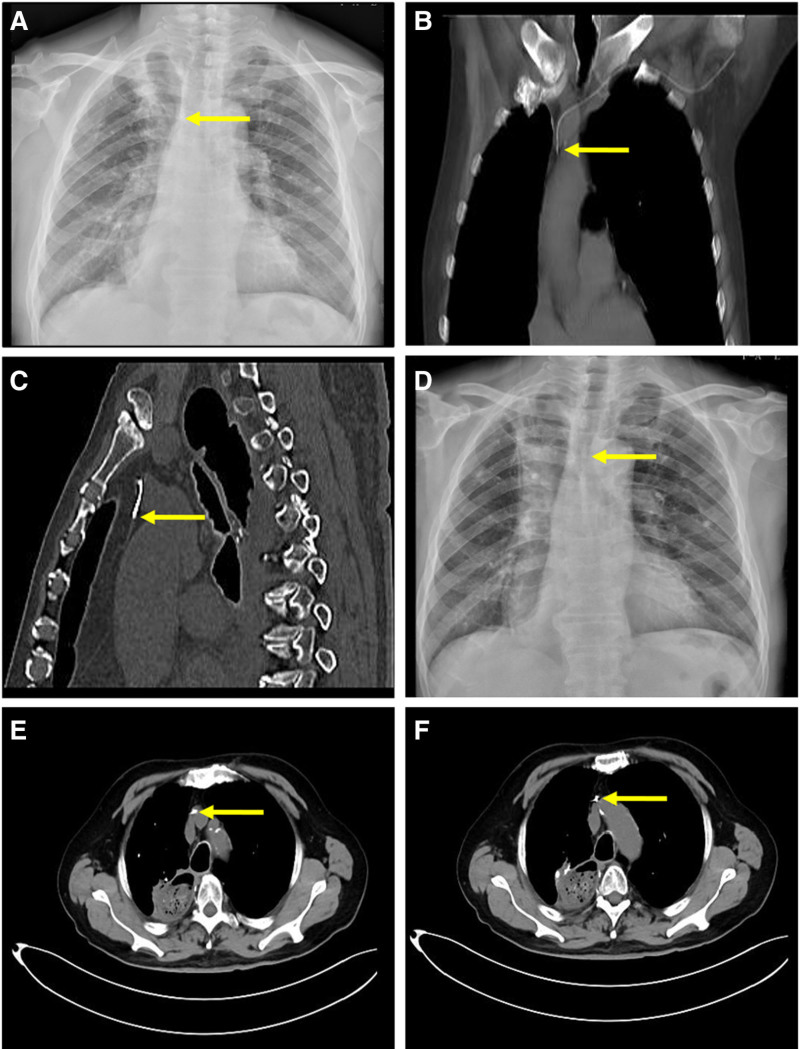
Radiographic information for Patient 1.
